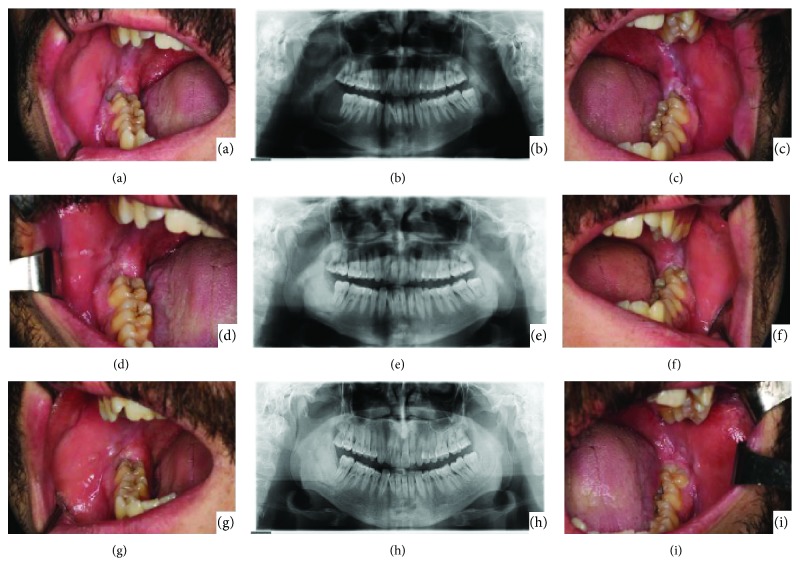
(a) Six months of follow-up: panoramic radiography of bilateral brown tumors. Notice to decreased radiolucency for the brown tumor in the bilateral sides of the mandible. (b) Six months of follow-up: intraoral appearance of the brown tumor (right side). (c) Six months of follow-up: intraoral appearance of the brown tumor (left side). (d) Second year of follow-up: panoramic radiography of bilateral brown tumors. Notice not to observe recurrence for brown tumors in the bilateral sides of the mandible. (e) Second year of follow-up: intraoral appearance of the brown tumor (right side). (f) Second year of follow-up: intraoral appearance of the brown tumor (left side). (g) Fourth year of follow-up: panoramic radiography of bilateral brown tumors. (h) Fourth year of follow-up: intraoral appearance of the brown tumor (right side). (i) Fourth year of follow-up: intraoral appearance of the brown tumor (left side). Notice to the left mandibular third molar eruption is almost completed.
